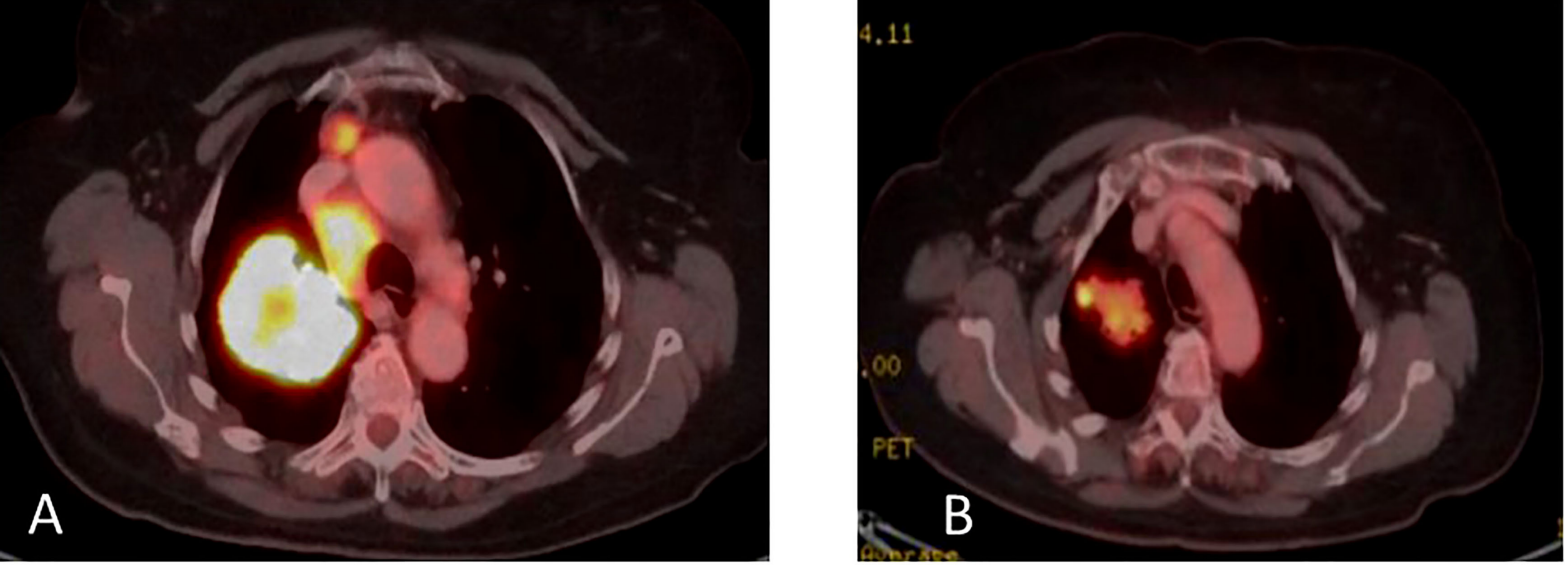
(A) Coronal PET-CT scan, from September 2017, show a large right lung mass with increased uptake of FDG-18. There is also ipsilateral hilar and mediastinal lymphadenopathy with positive PET uptake, contributing to classification as unresectable, stage 3B NSCLC. (B) January 2018, following induction erlotinib and sequential definitive radiation therapy 72 grays (Gy) indicating good partial response.
